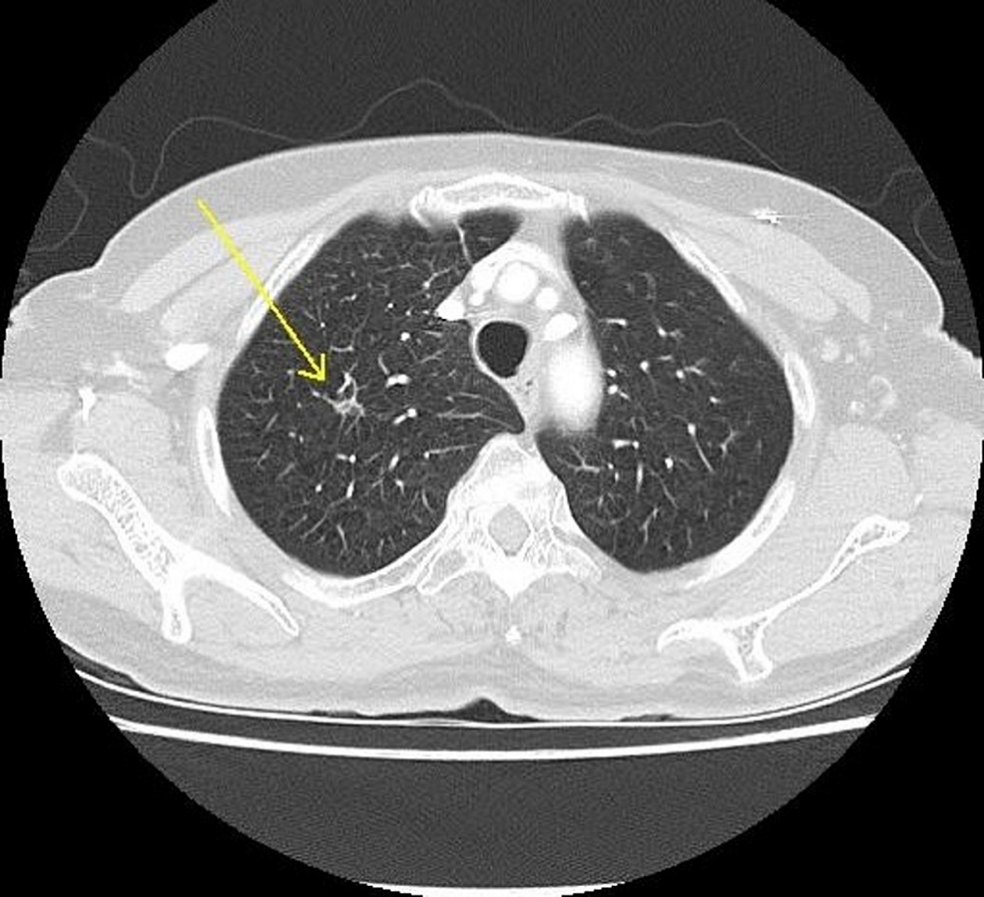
CT chest lung window showing stable lung field with a resolution of a prior nodule on 04/26/2021
